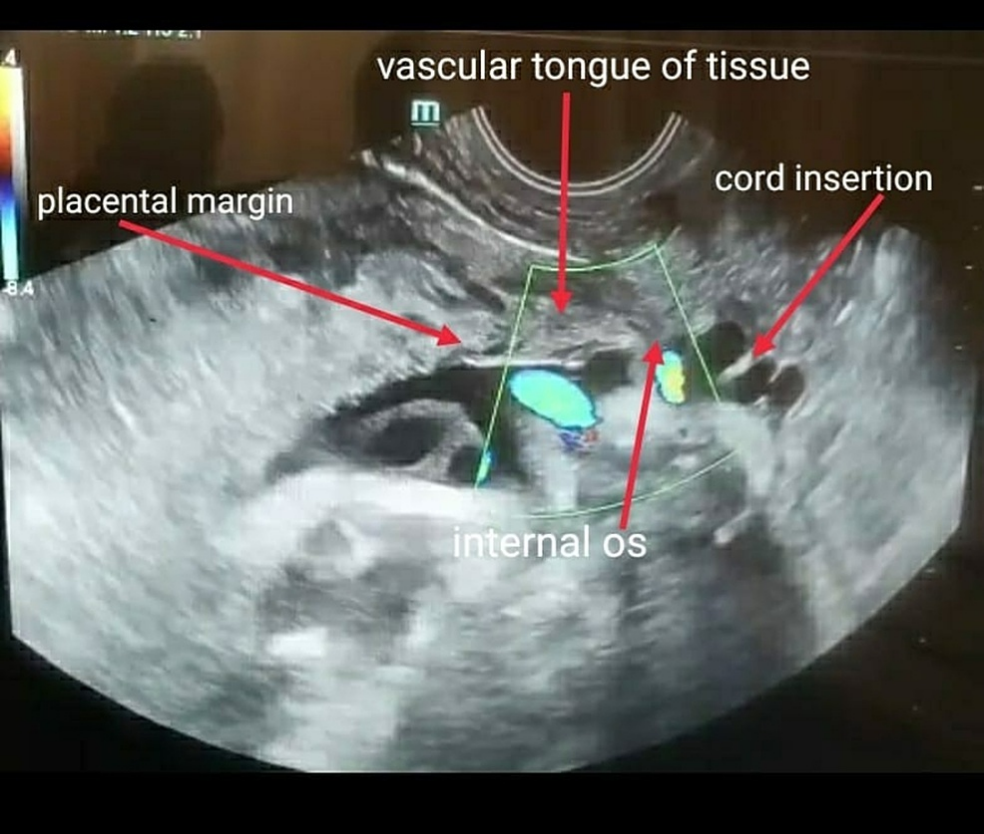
Prenatal ultrasound showing vasa previa.
